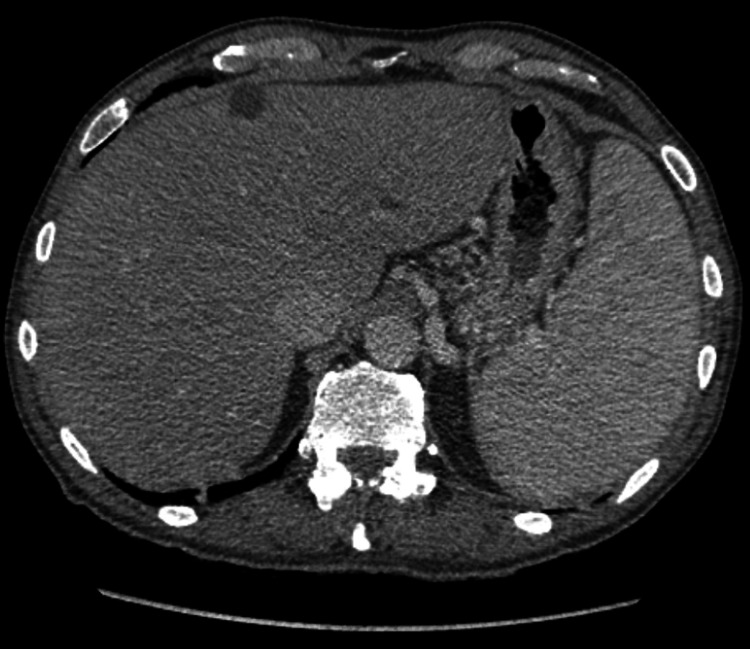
Case two: computed tomography of the abdomen and pelvis. Findings compatible with portal venous hypertension including splenomegaly and multiple collaterals.
